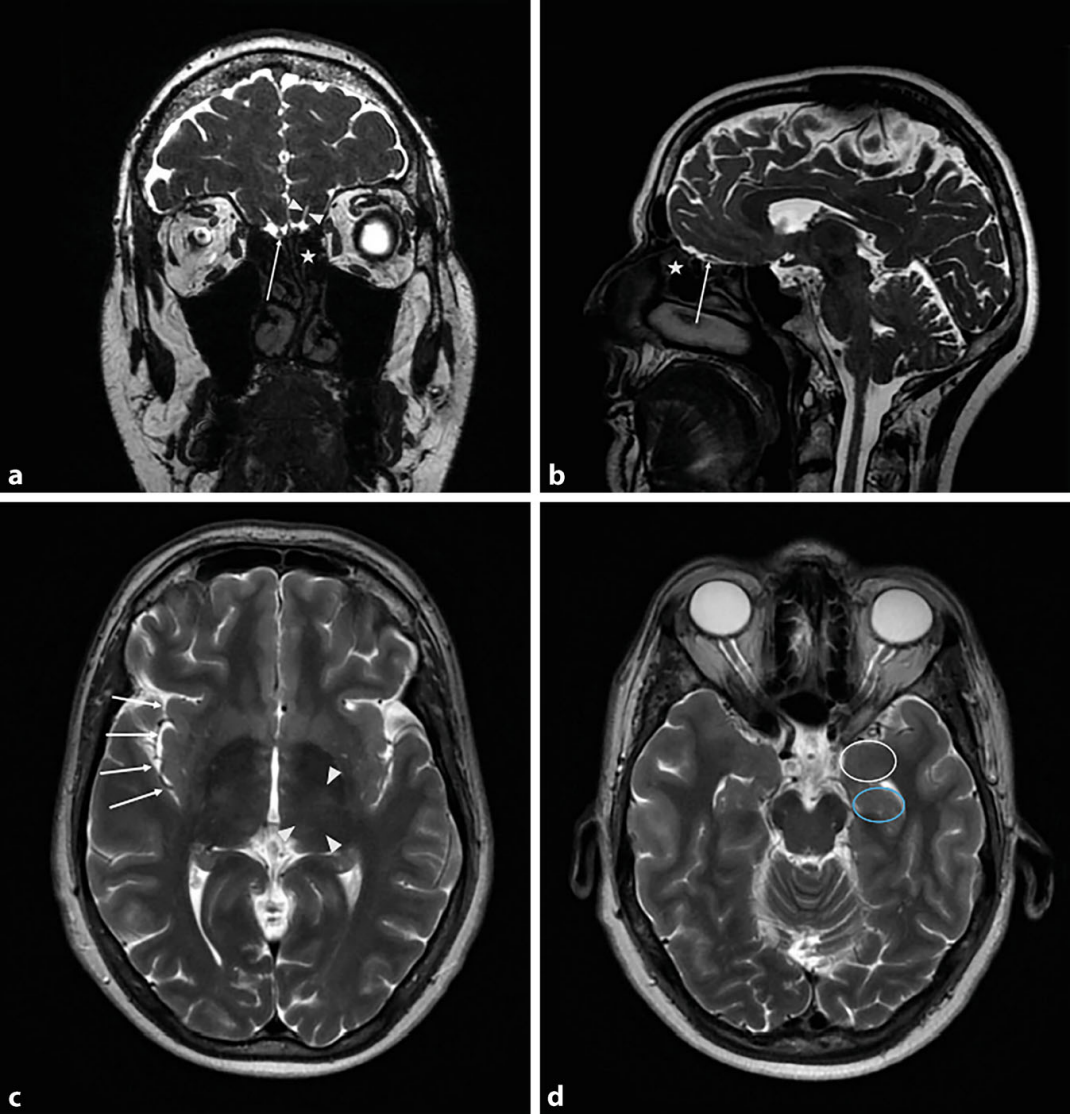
a Magnetic resonance imaging (MRI) of the skull; coronal T2-weighted image. Olfactory bulb (*arrow*), olfactory sulcus (*triangles*), olfactory mucosa (*star*). b MRI of the skull; sagittal T2-weighted image. Olfactory bulb (*arrow*), olfactory mucosa (*star*). c MRI of the skull; axial T2-weighted image. Insula (*arrows*), thalamus (*triangles*). d MRI of the skull; axial T2-weighted image. Amygdala (*white circle*), hippocampus (*blue circle*)
